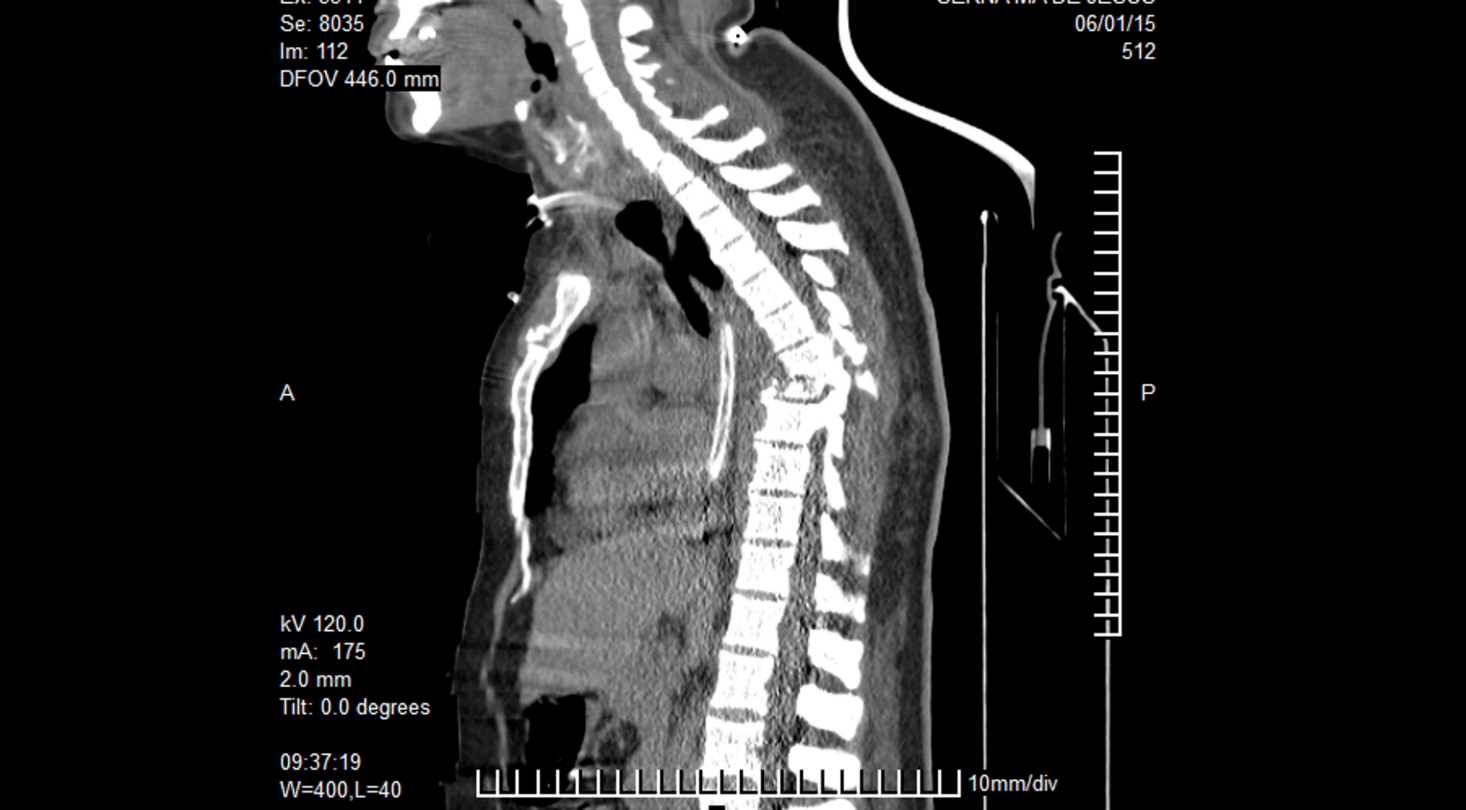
Case 1 CT scan midline sagittal cut image showing loss of continuity of the vertebral column at the level of the T6-T7 vertebrae.
